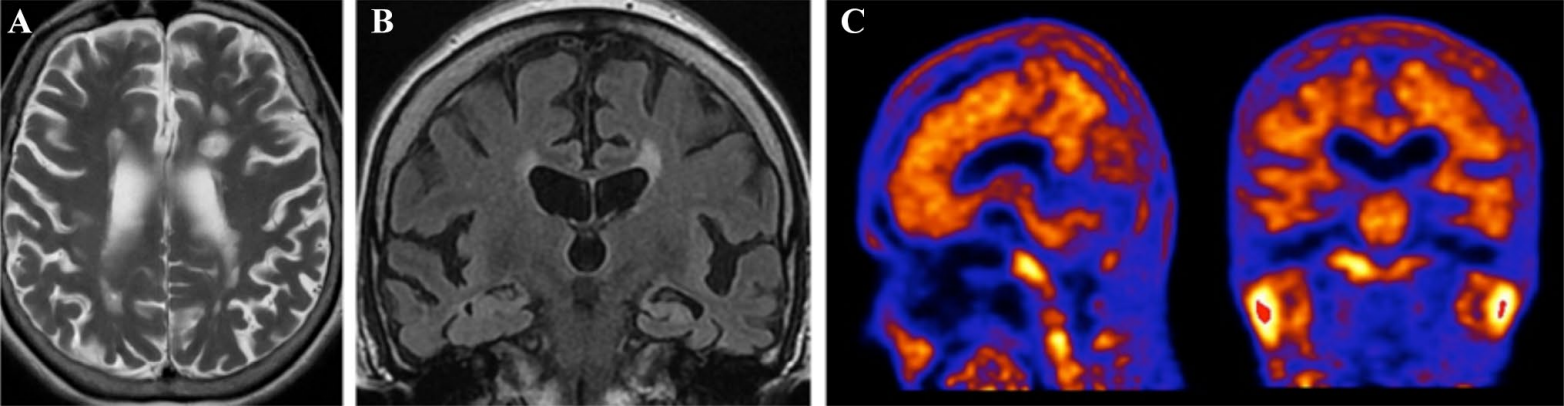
Case 1. Axial T2-weighted (a) and coronal FLAIR (b) images through the brain showing typical MS lesions in the periventricular white matter, perpendicular to the ependymal surface. Note generalised neuroparenchymal loss, with relative sparing of the temporal lobe white matter (left mesial temporal atrophy score is 2; right mesial temporal atrophy score is 1). Sagittal and coronal amyloid PET images (c) demonstrating generalised increased activity within the cortical grey matter in all lobes with complete loss of grey–white differentiation consistent with widespread amyloid deposition
